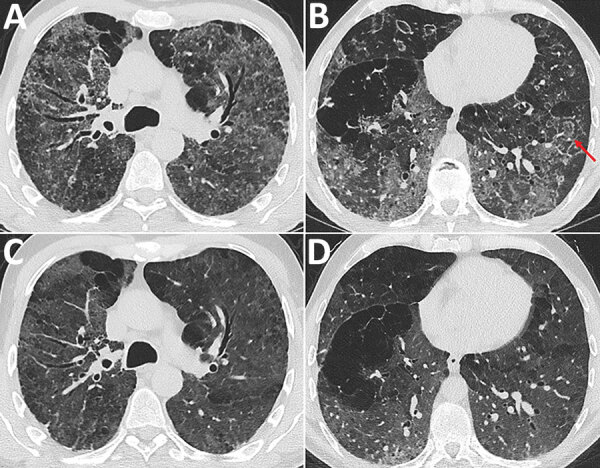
Chest computed tomography (CT) images at the level of the upper third and the lower third of the lung in a patient with pulmonary histoplasmosis, Switzerland. A, B) Initial CT shows diffuse reticulonodular pattern with ground glass opacifications, predominantly located in the upper two thirds of the lungs, and several areas with reverse halo signs (red arrows). C, D) Follow-up CT scan exhibited reduced ground-glass opacities and a regression of the micronodules. The reversed halos showed complete regression. CT, computed tomography.
